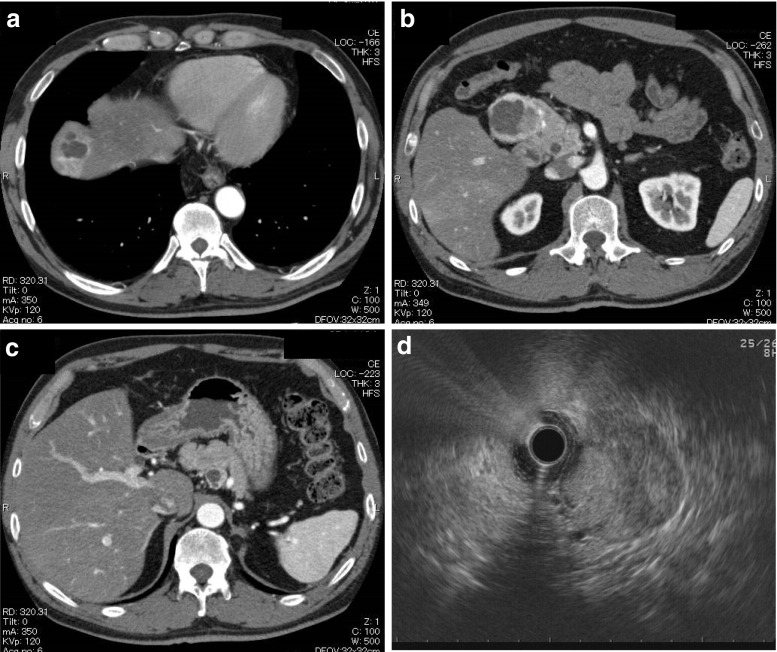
Imaging studies. a–c Dynamic contrast-enhanced CT scan during the artery phase showed a well-enhanced mass with cystic component in an anterosuperior segment (S8) of the liver (a), the head of the pancreas (b), and the posterior portion of the body of the pancreas (c). d Endoscopic ultrasound (EUS) showed a 4.1-cm hypoechoic and well-demarcated mass in the parenchyma of the pancreatic head
